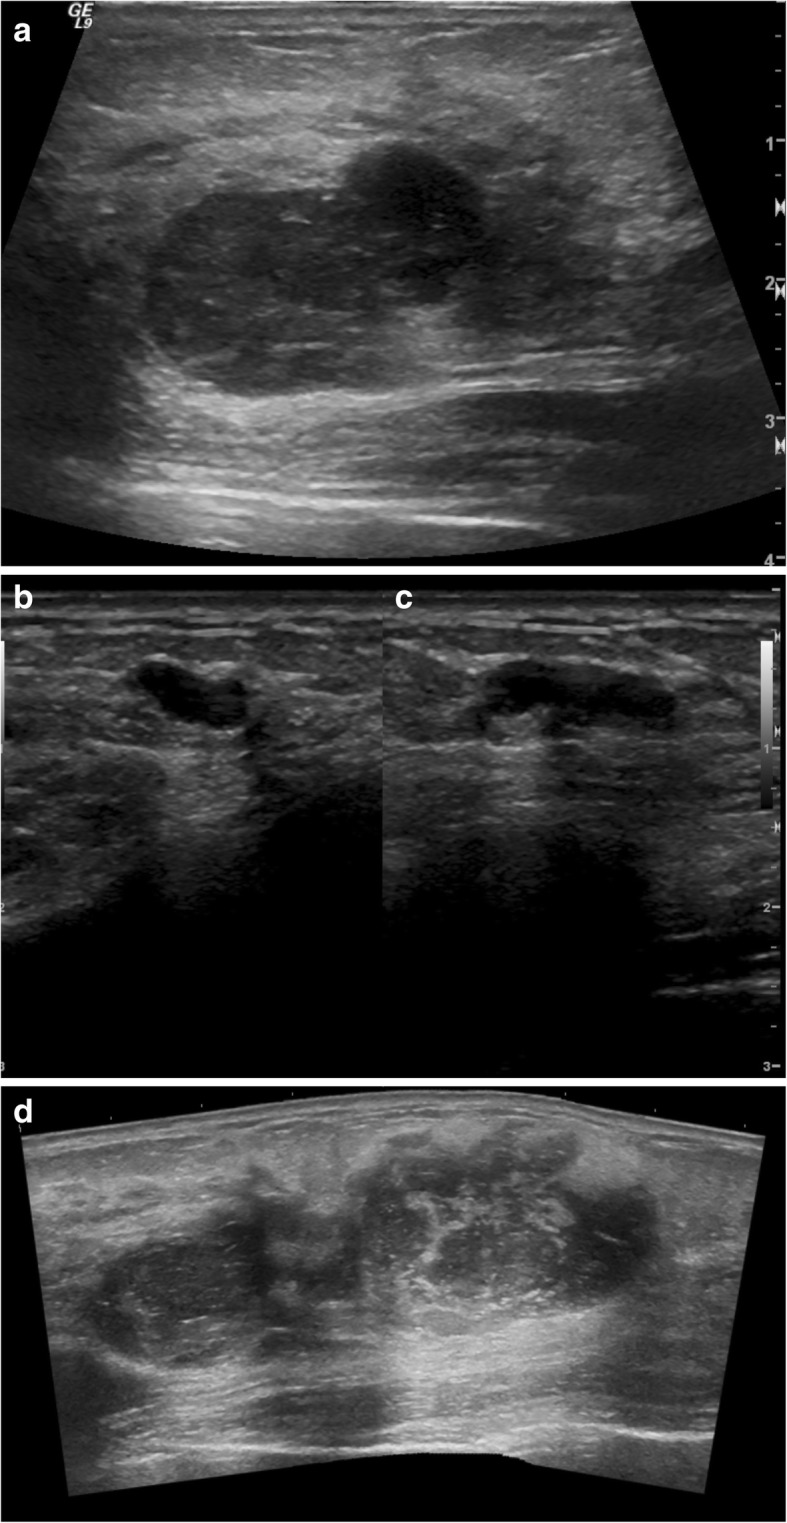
a Ultrasound image of left breast antiradial plane at 1:00 position 9 cm from the nipple demonstrates a mixed cystic and solid, irregularly shaped, hypoechoic, and heterogeneous mass with ill-defined margins and areas of posterior acoustic enhancement. Overall lesion size is 3.7 × 2.2 × 4.0 cm. b Ultrasound image of left breast antiradial plane at 12:00 position 8 cm from the nipple demonstrates a predominately solid, lobulated, hypoechoic, and heterogeneous mass with smooth margins and only minimal posterior acoustic enhancement. Overall lesion size is 3.4 × 1.8 × 2.7 cm. c Ultrasound image of left breast split screen antiradial (left) and radial (right) planes in 2:00 position 6 cm from the nipple demonstrates a predominately solid, lobulated, homogeneous, and hypoechoic mass with smooth, well-defined margins and areas of posterior acoustic enhancement. Overall lesion size is 0.8 × 0.4 × 1.2 cm. d Ultrasound image of the left breast with panoramic view shows the lesions shown in a and b may coalesce into a single larger lesion. This coalescent lesion is congruent with the pathologic findings of an 8.8-cm lesion
